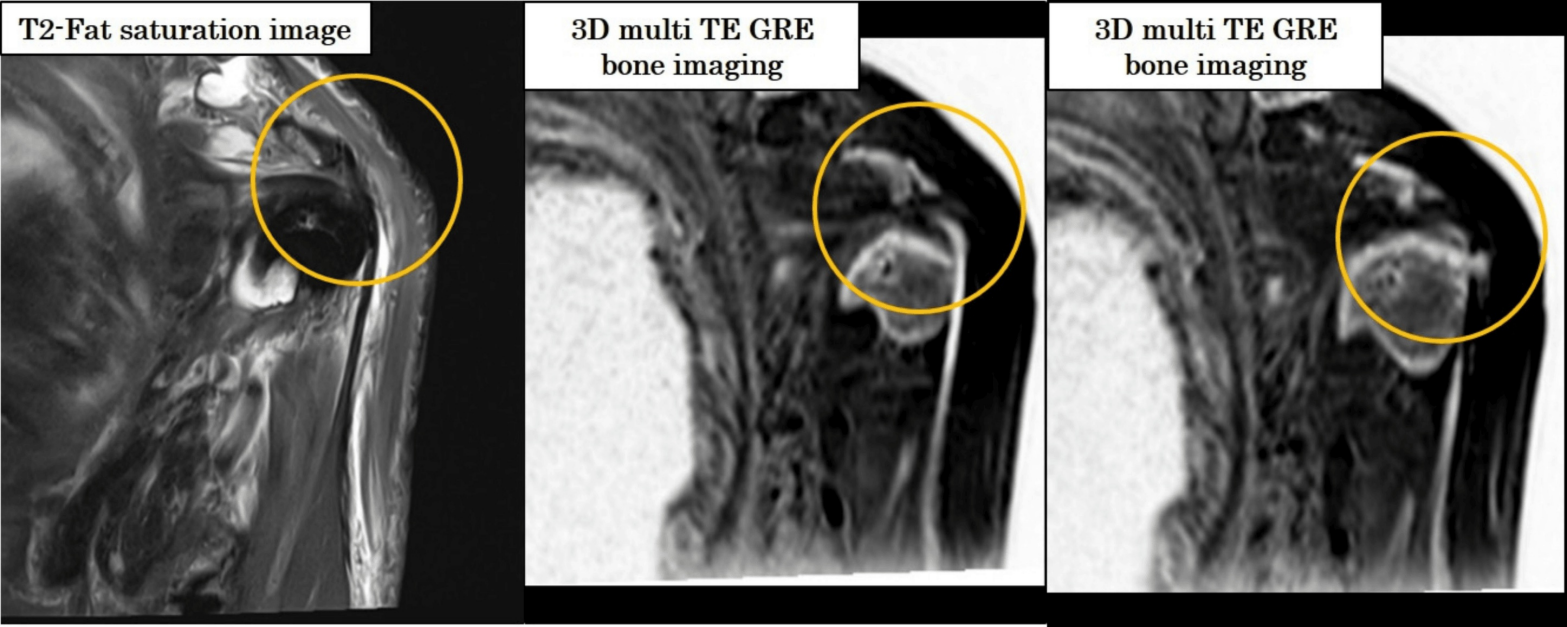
Long head of the biceps tendon attachment CT-like MRI delineates the attachment site despite fluid signal interference.
